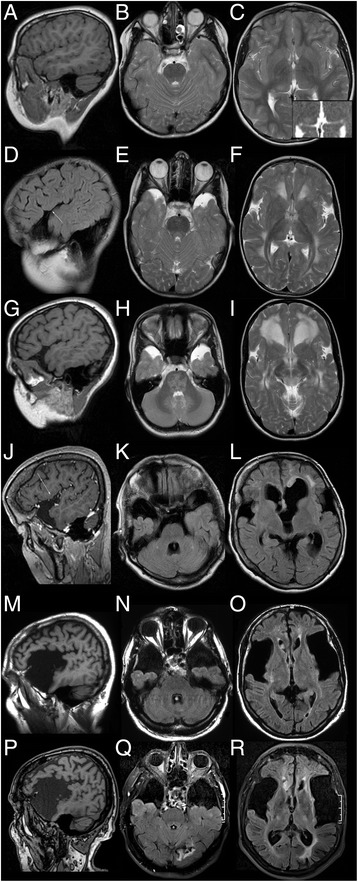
Normal frontotemporal anatomy and examples of frontotemporal hypoplasia. a-c Normal Sylvian fissure and anterior temporal CSF spaces in *control patient c2*. NB asymmetric thalamus with focal T2-hyperintensity and facilitated diffusion (inset: ADC map). d-i Mild hypoplasia in *late-onset patient p1* not extending dorsally beyond the pars opercularis (*arrow* in d) of the inferior frontal gyrus and remaining unchanged between first MRI at 8.6 years (d-f) and last follow-up MRI at 15.7 years (g-i). NB T2-hyperintensity of pontine white matter and dentate nucleus (H). j-l Moderate hypoplasia involving the subcentral gyrus in *late-onset patient p6* (*arrow* in j). NB subependymal nodules and focal, asymmetric *white* matter changes (l). m-r Widely open Sylvian fissure and massively widened anterior temporal CSF spaces in *late-onset patient p7* unchanged between MRIs at 61 and 73 years. NB large, subependymal, FLAIR-hyperintense nodule in right frontal horn (o, r; also Fig. 3). (*T1w:*
